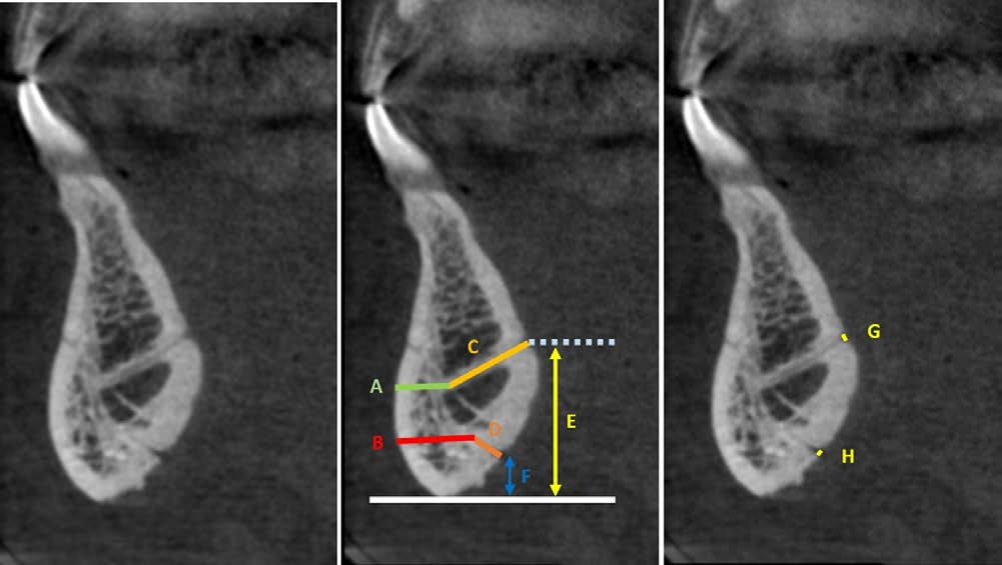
Dimensional measurements on cross-sectional mandibular CBCT images. (A and B) Distance from the deepest point of canal to the buccal cortex (Hhor). (C and D) Length of the canal (Lc). (E and F) Distance from the canal orifice to the mandibular inferior cortex level (Hinf). (G and H) Diameter of the exit orifice (Df). CBCT = cone beam computed tomography.
